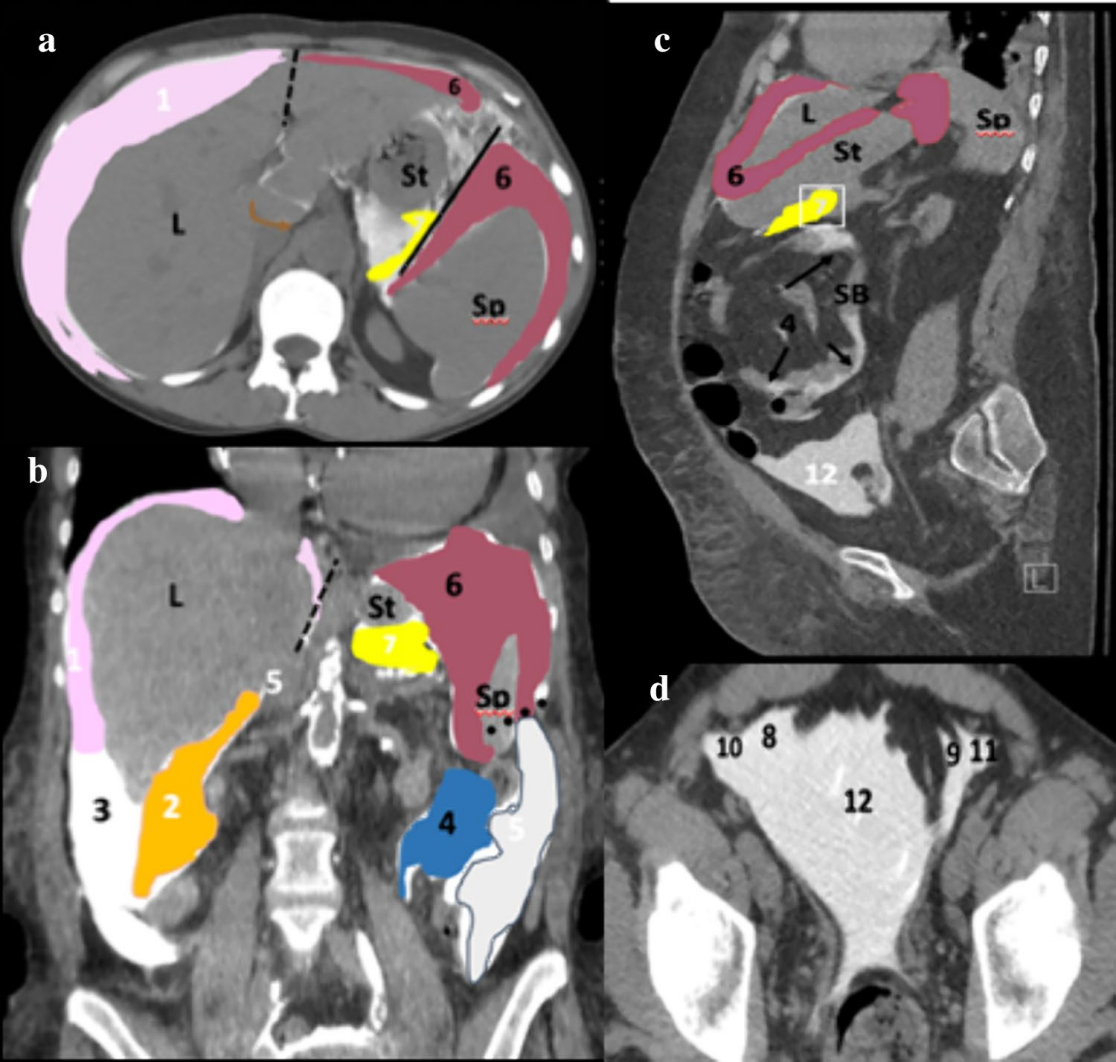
Cases depicting the peritoneal spaces in patients while evaluating for a peritoneal dialysis leak. Axial CT section (a), coronal CT section (b), and sagittal CT section of the abdomen show contrast within peritoneal recesses. Important peritoneal anatomy includes the right subphrenic recess (1, pink), right subhepatic recess (2, orange), right paracolic gutter (3, white), left inframesocolic recess (4, blue), left paracolic gutter (5, light gray), and left subphrenic recess (6, dark magenta). Ligaments depicted include the phrenicocolic ligament (black dotted line), falciform ligament (black dashed line), and gastrosplenic ligament (black solid line). The foramen of Winslow (brown arrows) provides the only passage between the greater and lesser sac (7, yellow). Inferiorly, pelvic contrast opacifies the right and left medial (8 and 9 respectively) and lateral (10 and 11 respectively) inguinal recesses, as well as the supravesical recess (12) in (c) and (d). Abbreviations include L-liver, Sp-spleen, SB-small bowel, and St-stomach
